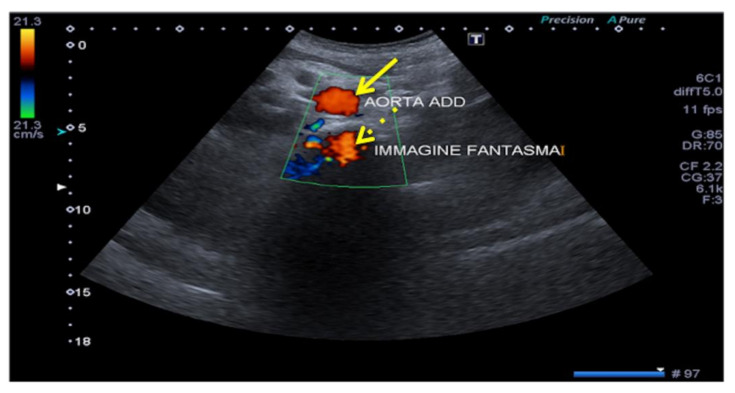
Aorta ghosting. Transverse mesogastric color-Doppler US image obtained at the level of the infra-renal aorta (arrow) shows aorta ghosting (dashed arrows) that projects backwards with the same color sign.
